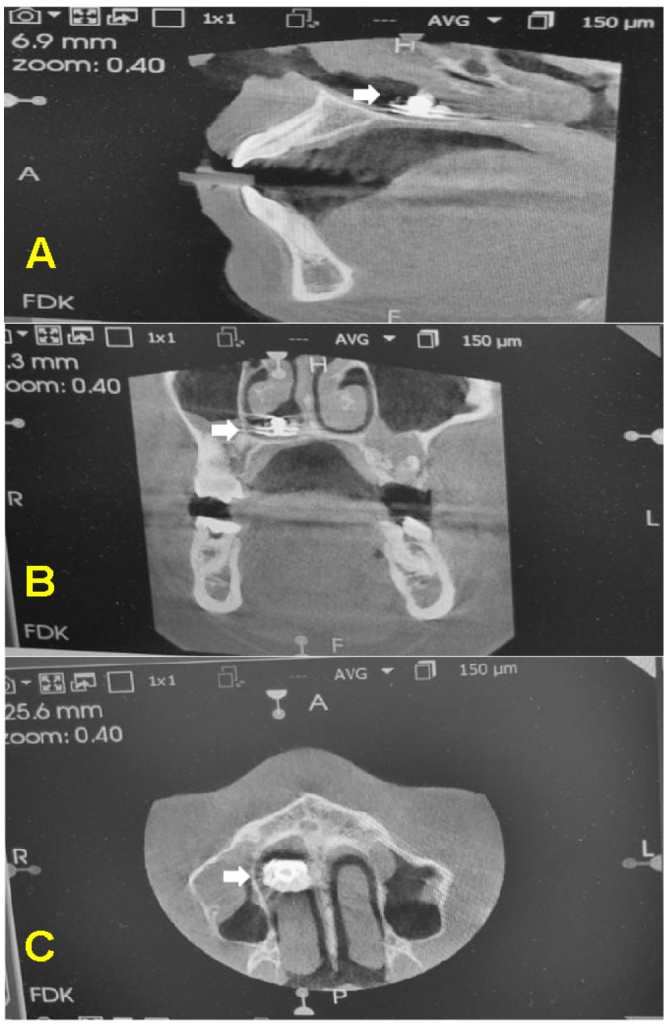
Cone beam computed tomography (CBCT) without contrast (A; Sagittal, B; coronal and C; Axial views) showing a well-defined, circular, hyperdense object located posteriorly *(arrows)*.
